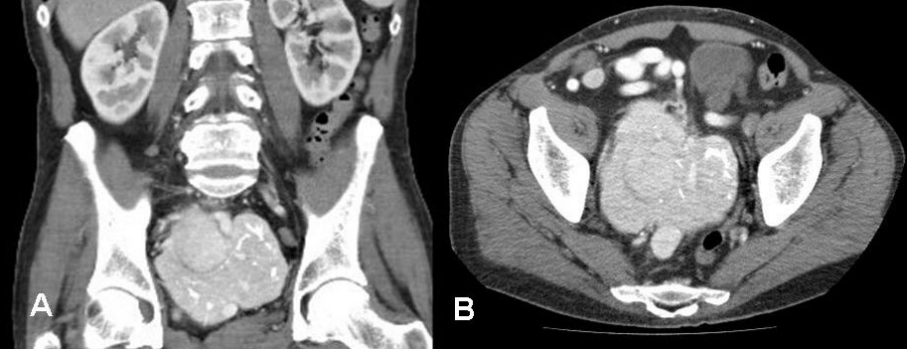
A). Preoperative CT scan of the abdomen showing a highly vascularized retroperitoneal tumor measuring 10 × 9.2 cm with intratumor calcifications. Right ureter dilatation (grade II), (coronal multiplanar reformation, MPR) B). The same tumor in axial orientation. No other tumor localization in the abdomen nor enlarged lymph nodes were detected.
